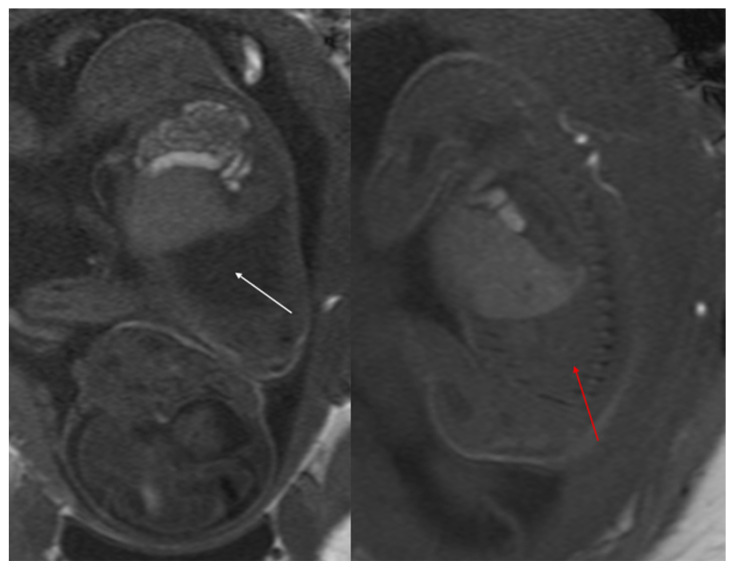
Sagittal T1w FLASH MRI sequence shows increased signal intensity in the left abnormal pulmonary parenchyma (white arrow) and the compressed signal of the right lung (red arrow). A differential diagnosis to consider is congenital lobar overinflation (CLO), where a more homogeneous signal intensity would typically be observed.
